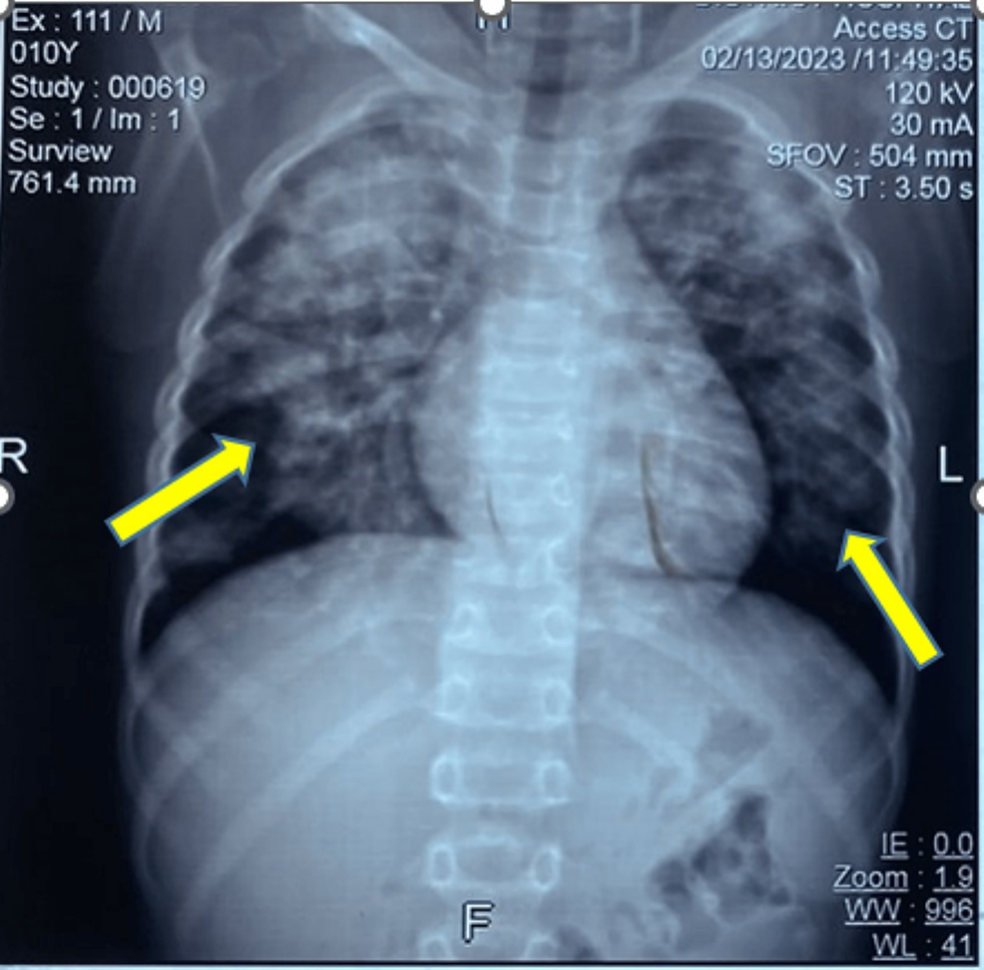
Computed tomography of the thorax (scout film) showing bilateral multifocal patchy hyperdensities.
